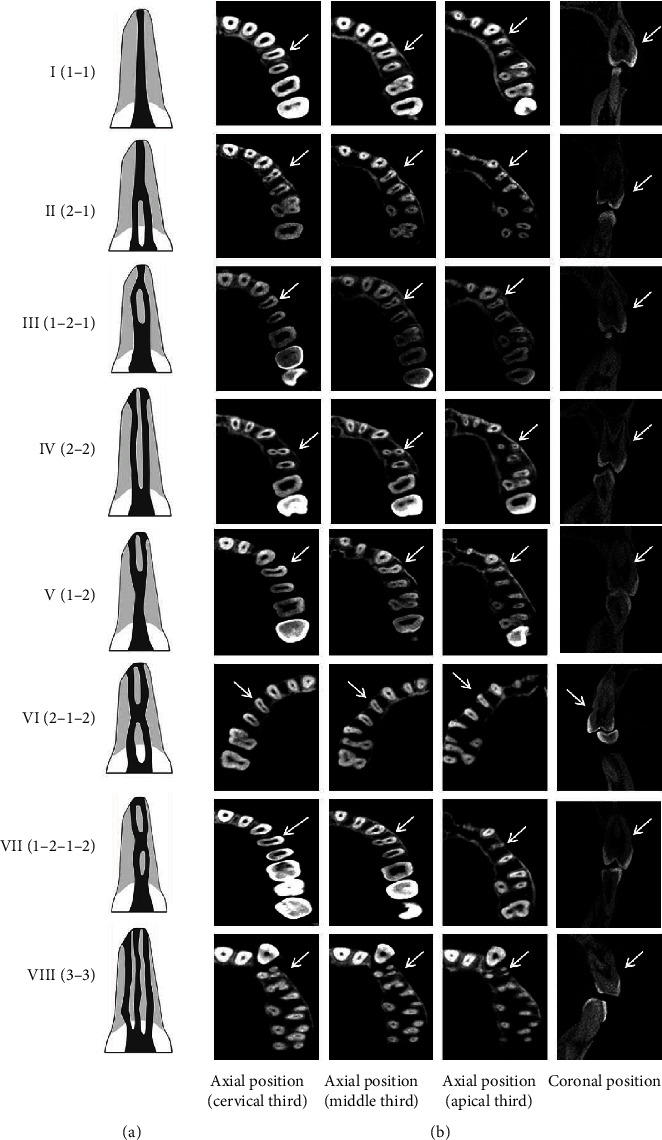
Classification of root canal system according to Vertucci's classification (a) and CBCT images showing root canal configuration of maxillary first premolars (b). Arrows indicate the examined teeth.
